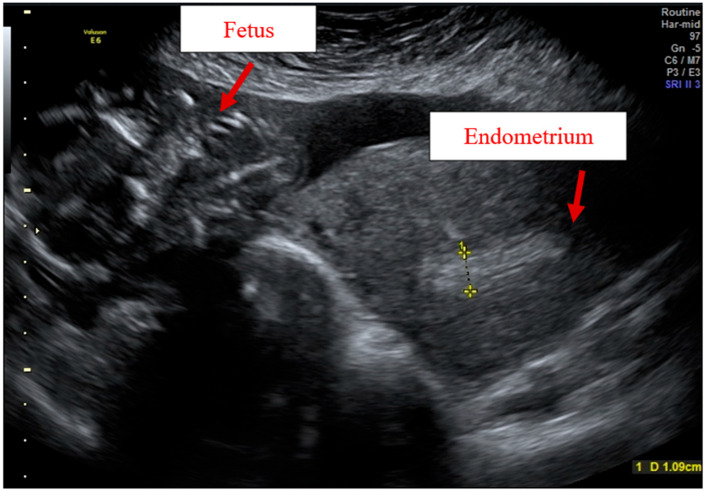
Ultrasound before laparotomy: fetus with amniotic sac completely expelled into the abdomen.
